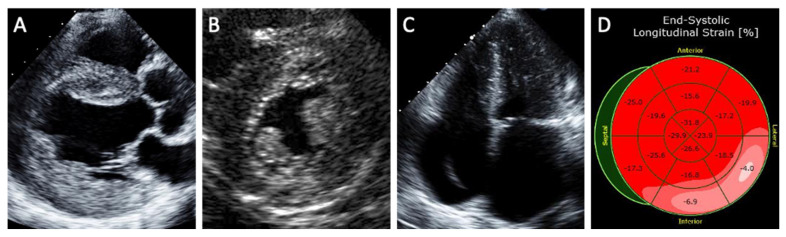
Structural abnormalities detected on echocardiography in patients with Fabry cardiomyopathy. (A) Parasternal long-axis view shows increased left ventricular wall thickness (the hallmark feature of Fabry cardiomyopathy) along with thickening of the aortic and mitral valves as well as aortic dilatation. (B) Parasternal short-axis view shows prominent papillary muscle and binary sign (hyperechogenic endocardial layer of glycolipid-enriched smooth muscle cells adjacent to a hypoechogenic subendocardial layer relatively devoid of glycolipids). (C) Severe biatrial enlargement with a device lead seen in the right-sided chambers for treatment of advanced conduction disease. (D) Reduced longitudinal strain in the basal inferolateral segment characteristic of Fabry cardiomyopathy.
